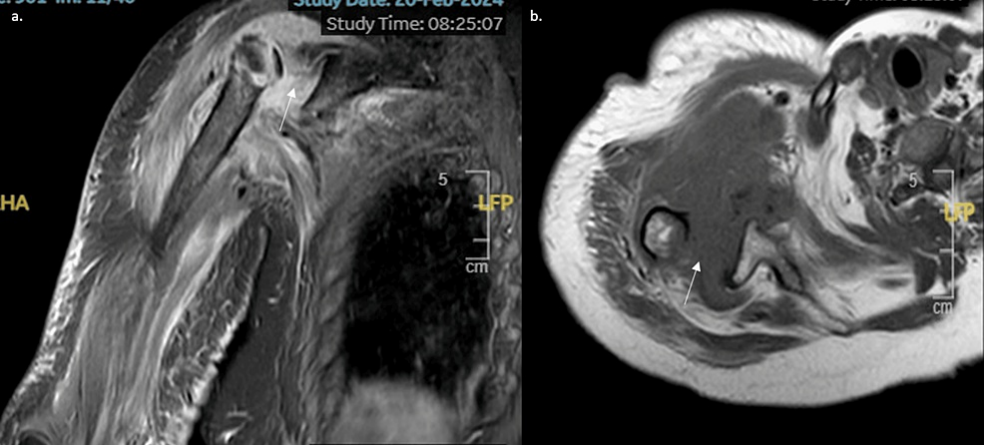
Magnetic Resonance Imaging radiographs prior to surgery a. Coronal b. Sagittal MRI view of the shoulder showing destruction of the shoulder joint (white arrows).
